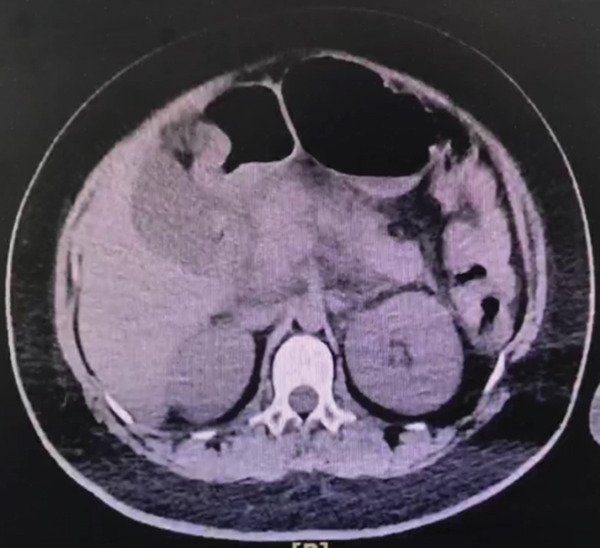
Abdominal CT showing pancreatic enlargement with marked peripancreatic inflammatory exudation, consistent with acute pancreatitis.
